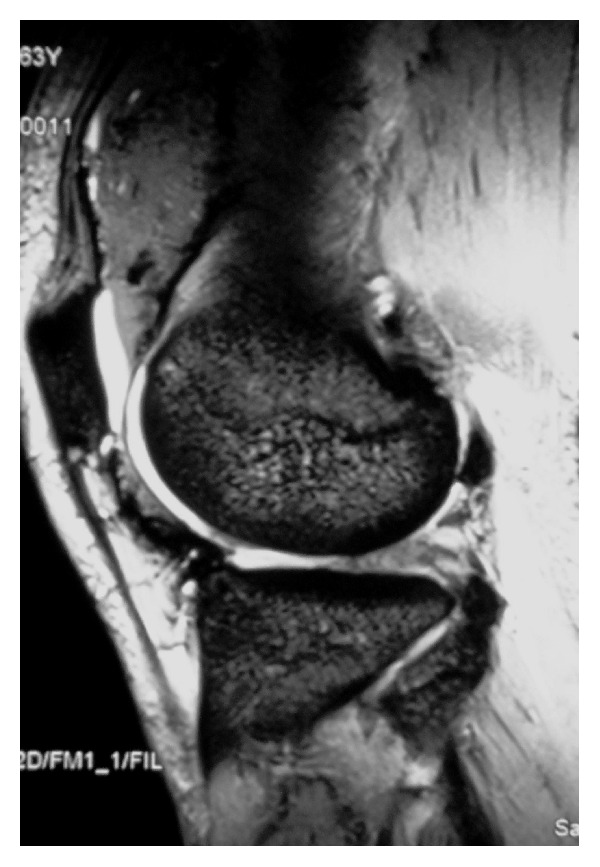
MRI of Case 1 (T2 weighted image). The posterior portion of the lateral meniscus is torn.
